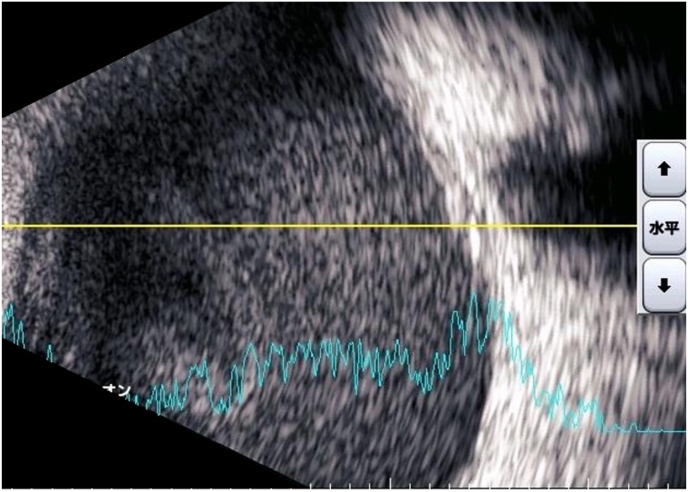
Ultrasound B-scan tomography of the right eye showed a reflection of dense vitreous opacity.
